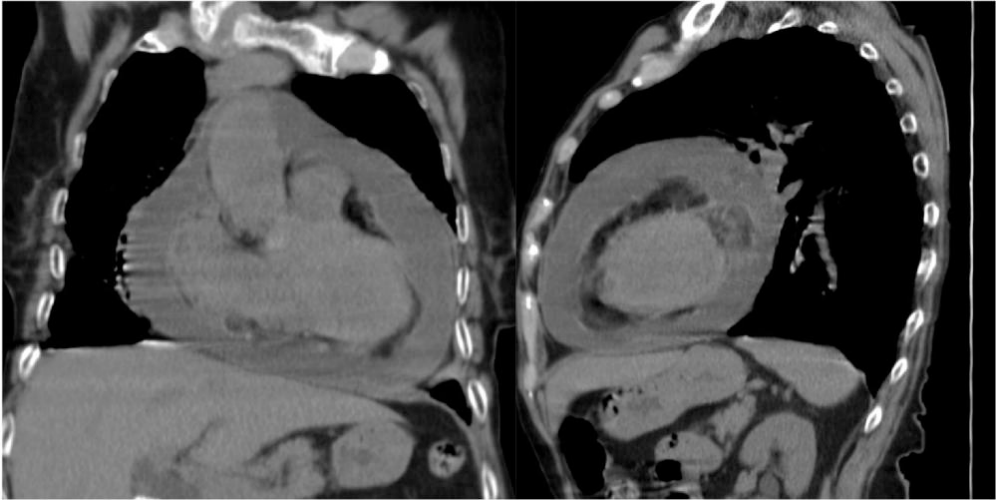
Pericardial effusion (thoracic computed tomography).
